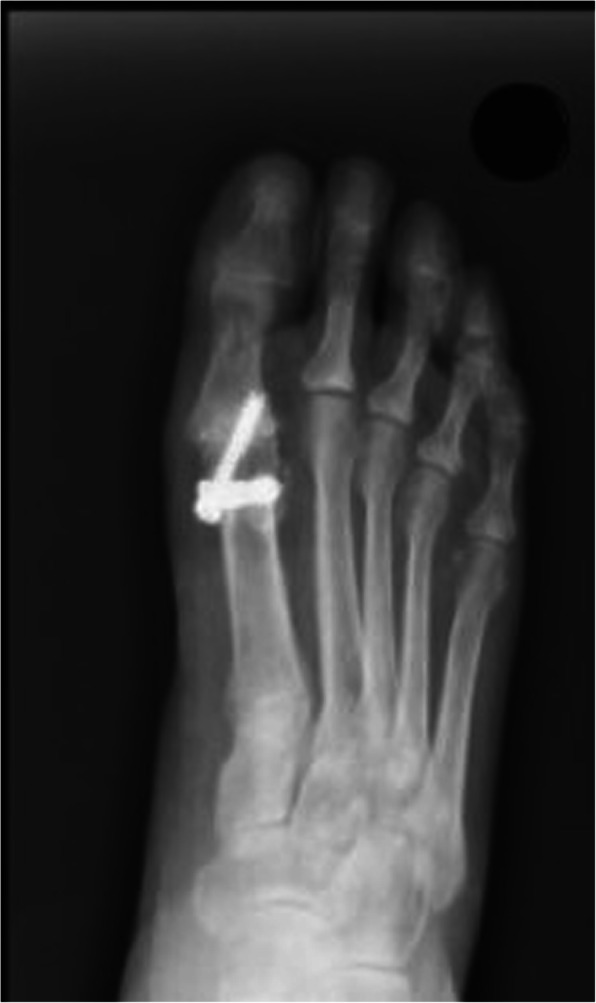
A standing X ray radiograph of a patients who was operated for a hallux rigidus with an IOFix implant. This patients suffered from a hardware protrusion. In this case a technique failure has led to the unwanted complication
